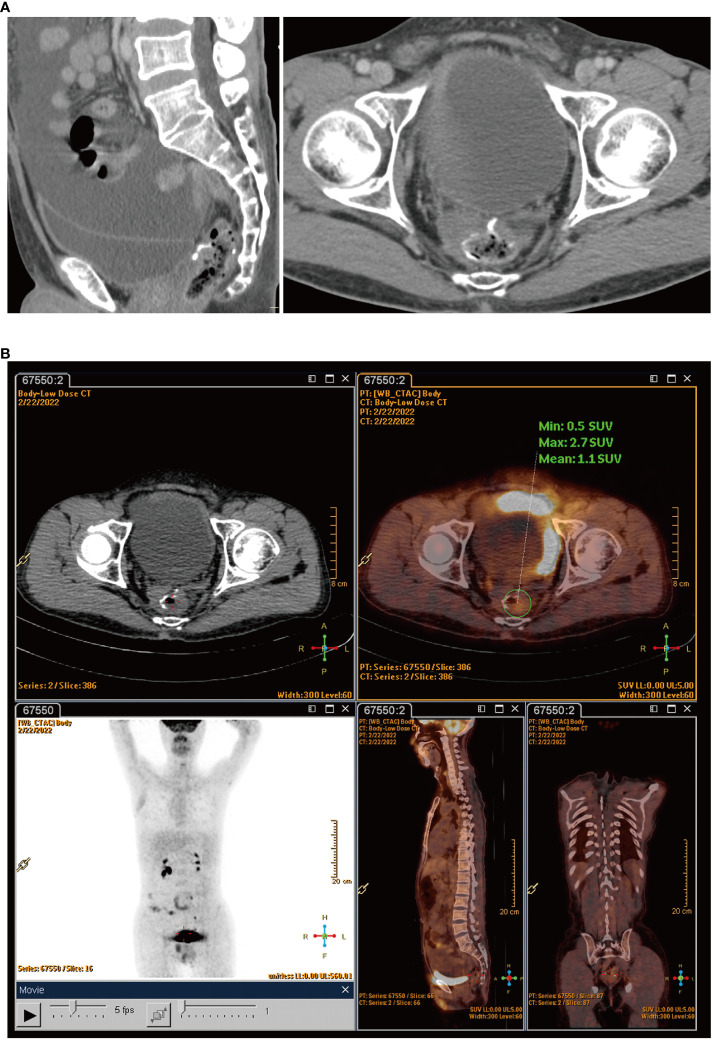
(A) Rescan of enhanced CT found a large amount of fluid in the abdominal cavity. (B) PET-CT scans showed uneven thickening of the peritoneum, omentum, and mesentery.
